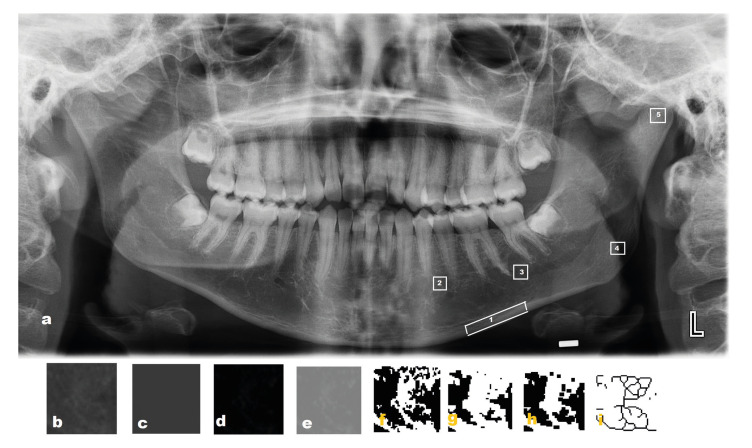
Panoramic radiograph with the five selected interest areas; 1: interest area on the cortical bone, 2: interest area of the premolar region, 3: interest area of molar region, 4: interest area of the angulus mandible, 5: interest area of condyle (a); cropped and duplicated image (b); a gaussian blurred image (c); a subtraction image (d); an added 128 image (e); binarization (f); erosion (g); dilation (h); and skeletonization (i).
